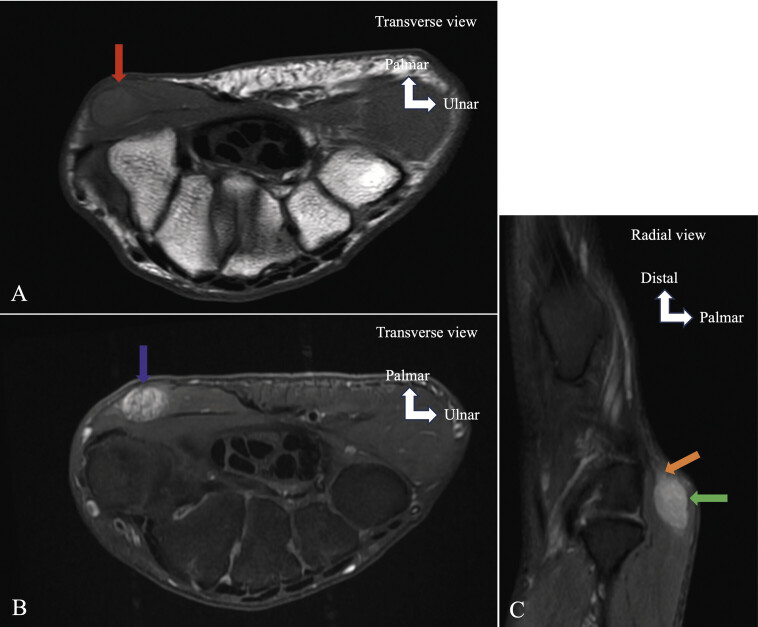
Schwannoma. Axial T1-weighted image
